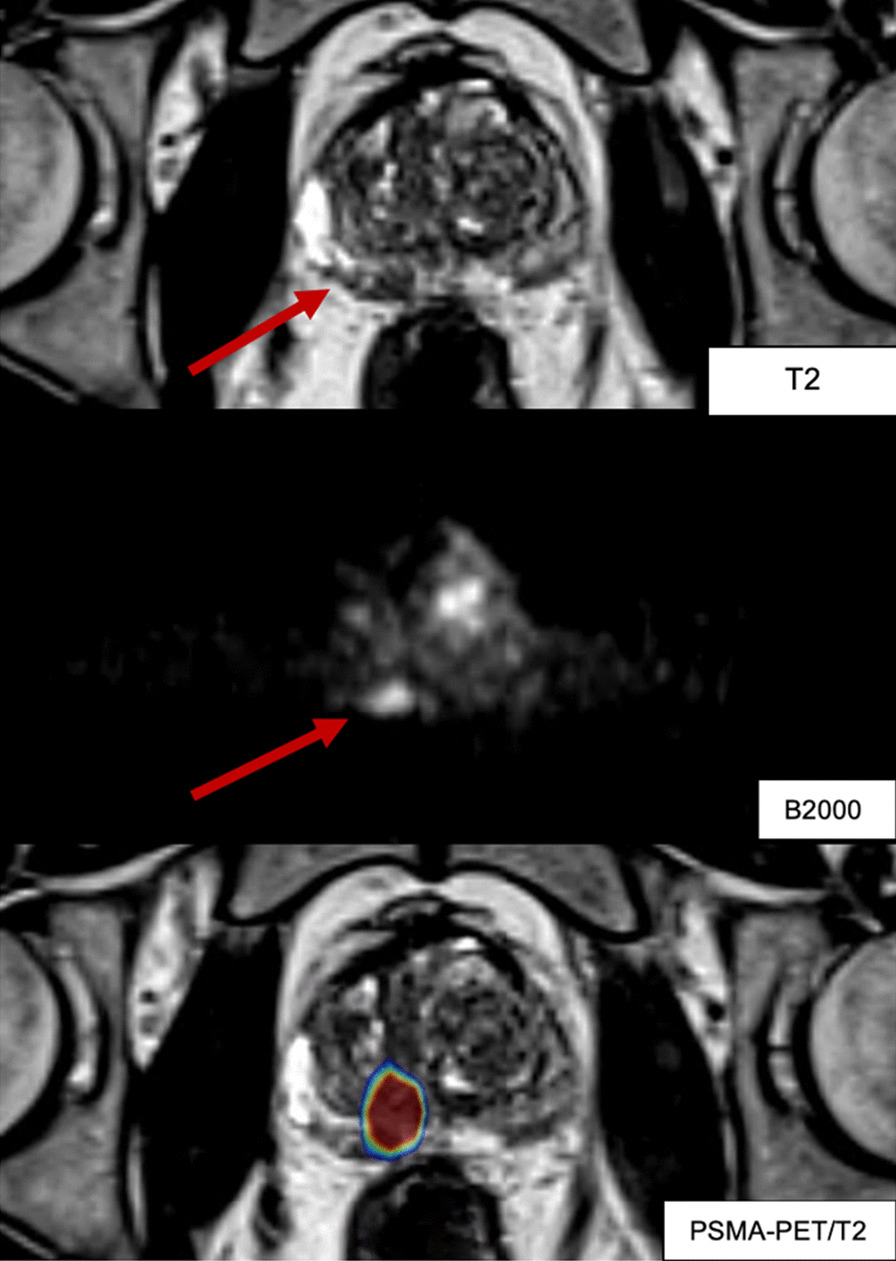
Images in T2, b2000 and PSMA-PET (PSMA-PET/CT registered to T2) images showing a GTV in the right peripheral zone of a patient planned for fBT
